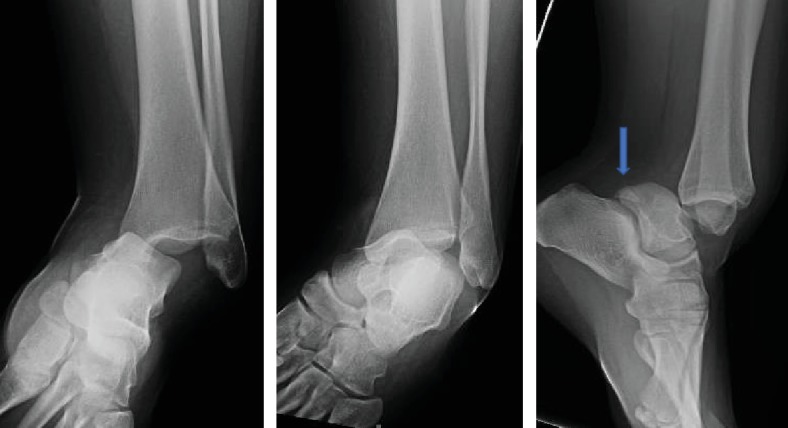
AP, lateral, and mortise radiograph demonstrating posteromedial ankle dislocation. The arrow on lateral view points towards double density over posterior talus representing displaced os trigonum.
